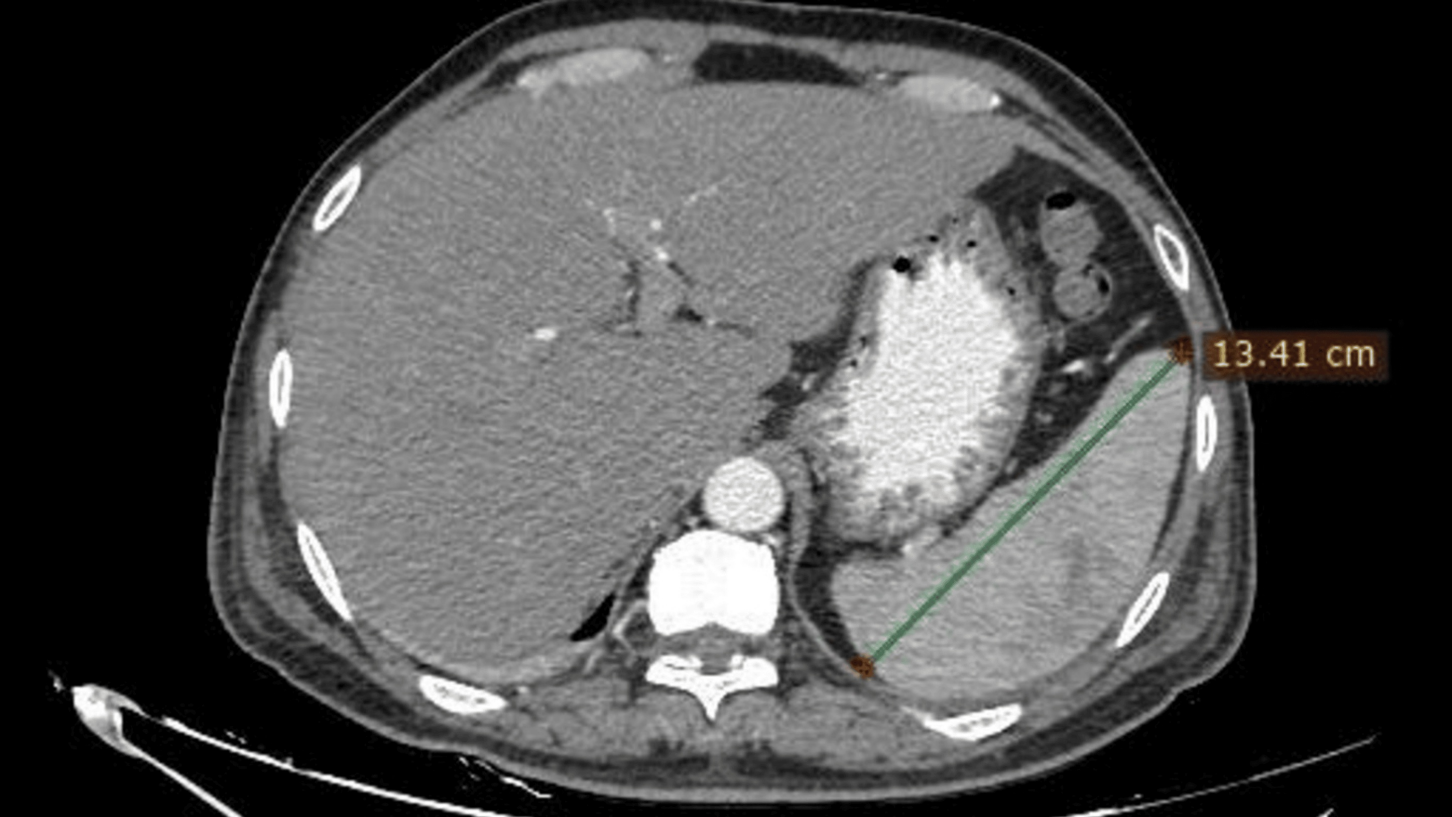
A CT scan of the abdomen shows splenomegaly, as represented by the green tracer above.
